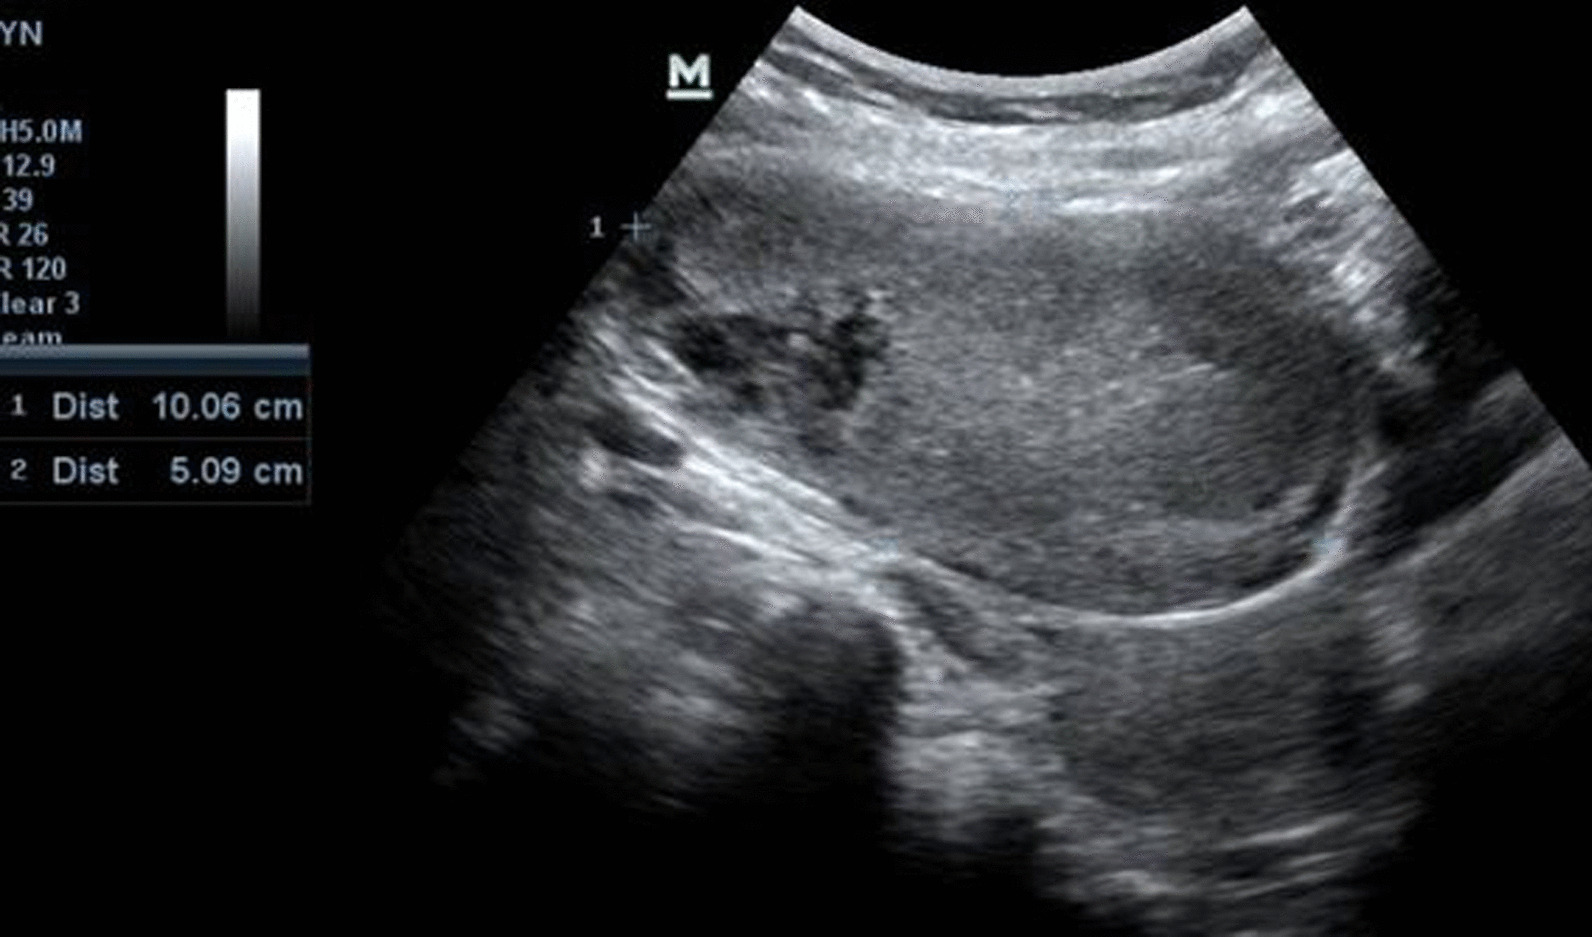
Ultrasonography image showing a well-defined cystic and solid lesion with areas of calcification
